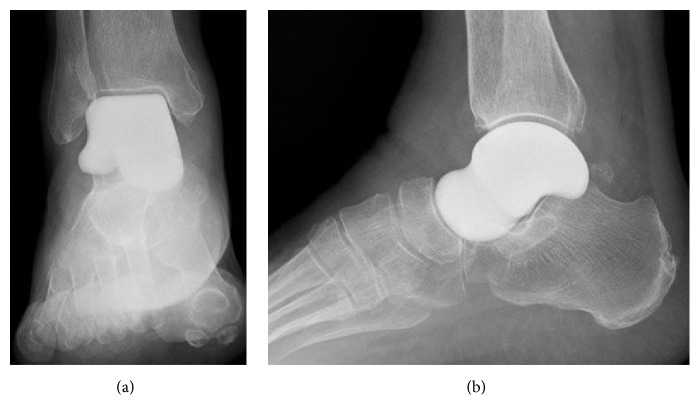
Postoperative radiographs showing the outcome of replacement of the talus with an alumina ceramic total talar prosthesis in anteroposterior (a) and lateral (b) views at 1 year and 6 months after surgery. There is good congruence between the talar prosthesis and the tibia, fibula, calcaneus, and navicular bone.
